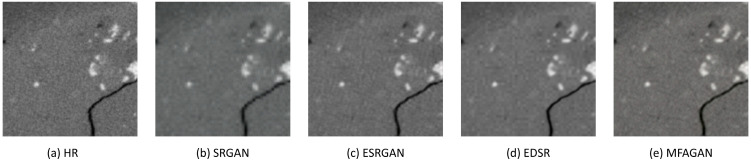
CT image reconstruction of conglomerate cores with different methods at ×4 magnification.
